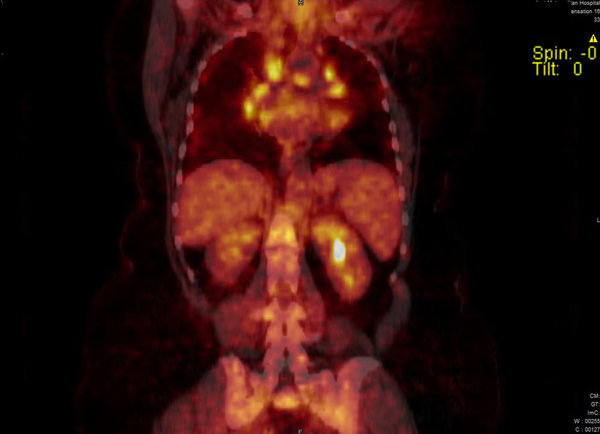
Positron emission tomography-computed tomography image showing hypermetabolic mediastinal lymph nodes.
